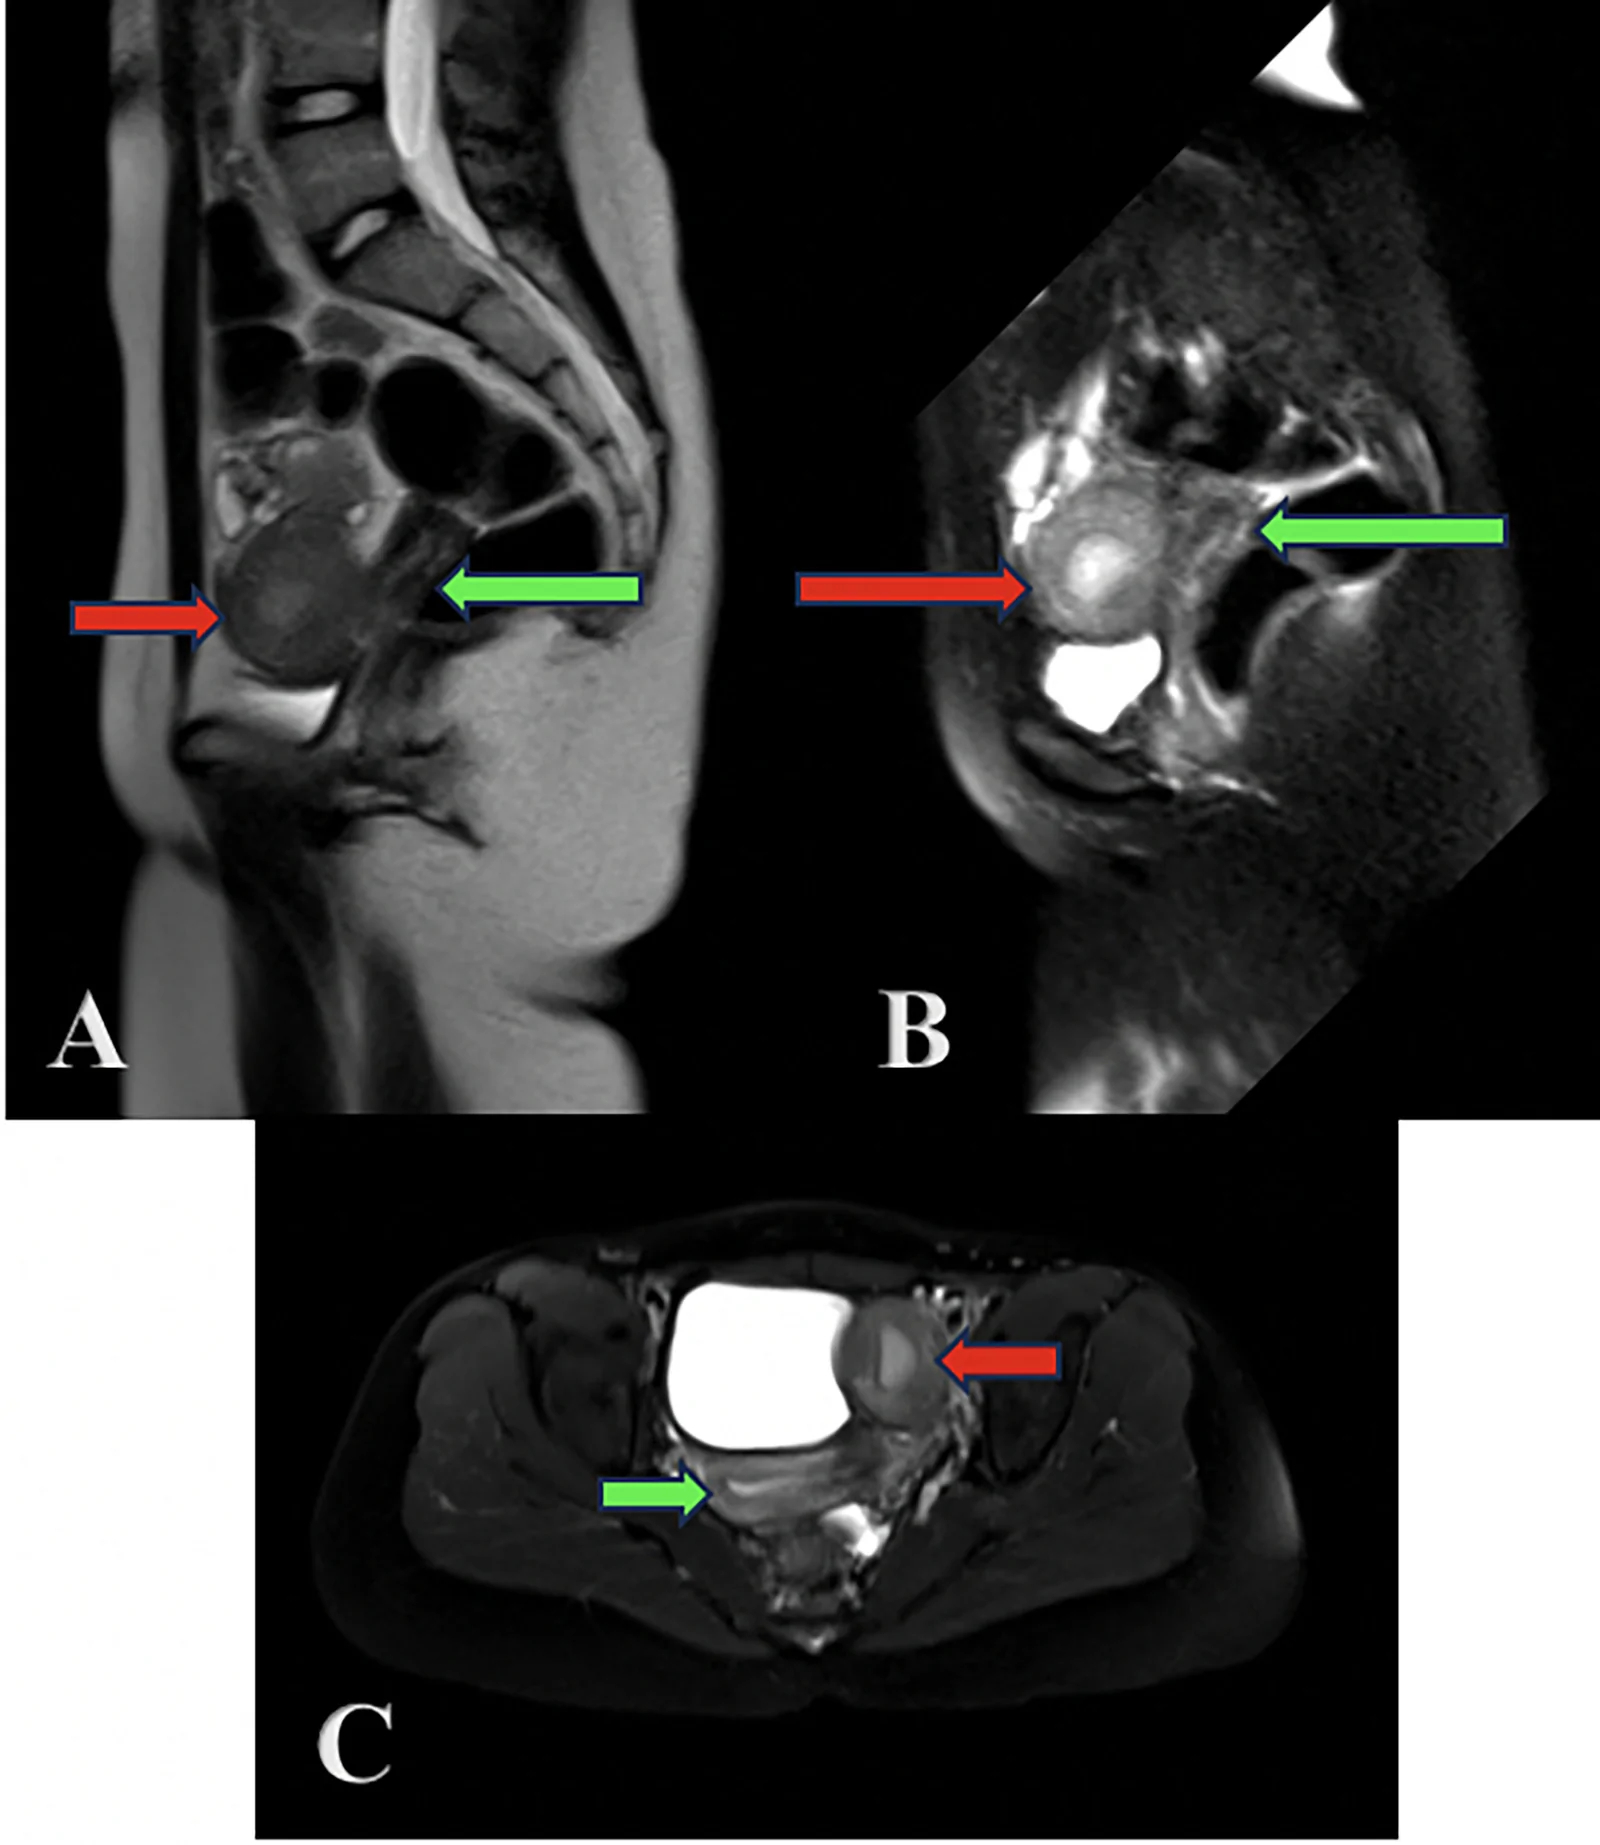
Pelvic MRI demonstrating features of Herlyn-Werner-Wunderlich syndrome with associated complications. (A) Sagittal T2-weighted image, (B) Sagittal T2-weighted fat-suppressed image, (C) Axial T2-weighted fat-suppressed image. All images demonstrate 2 separate uterine bodies consistent with uterus didelphys. The posterior structure represents the right uterus with a normal cervix and patent hemivagina (marked with a green arrow), without evidence of obstruction. The anterior enlarged structure represents the left uterus (marked with a red arrow), demonstrating absence of a visible cervix (cervical atresia), accompanied by marked distention of the endometrial cavity and no communication with the vaginal canal, consistent with obstructed hemivagina.
